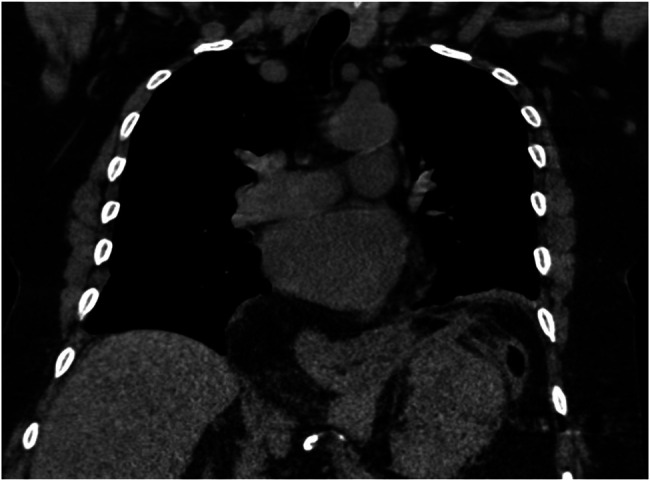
Computed tomography of the chest without contrast: performed 4 years earlier for another separate indication with incidental finding of abdominal mass captured in upper abdominal cuts of imaging.
